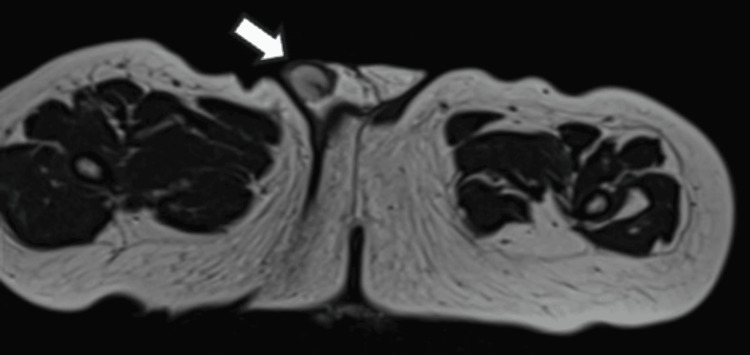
Axial T2-weighted MRI Right testicle (arrow) at the level of the scrotal sac
